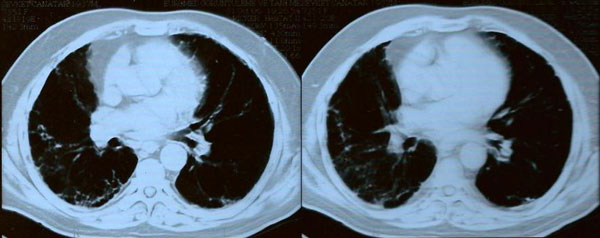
Repeat HRCT of patient performed after two months discontinuation of PEG-IFN and ribavirin with systemic steroid therapy, showing improvement of the ground glass opacities.
